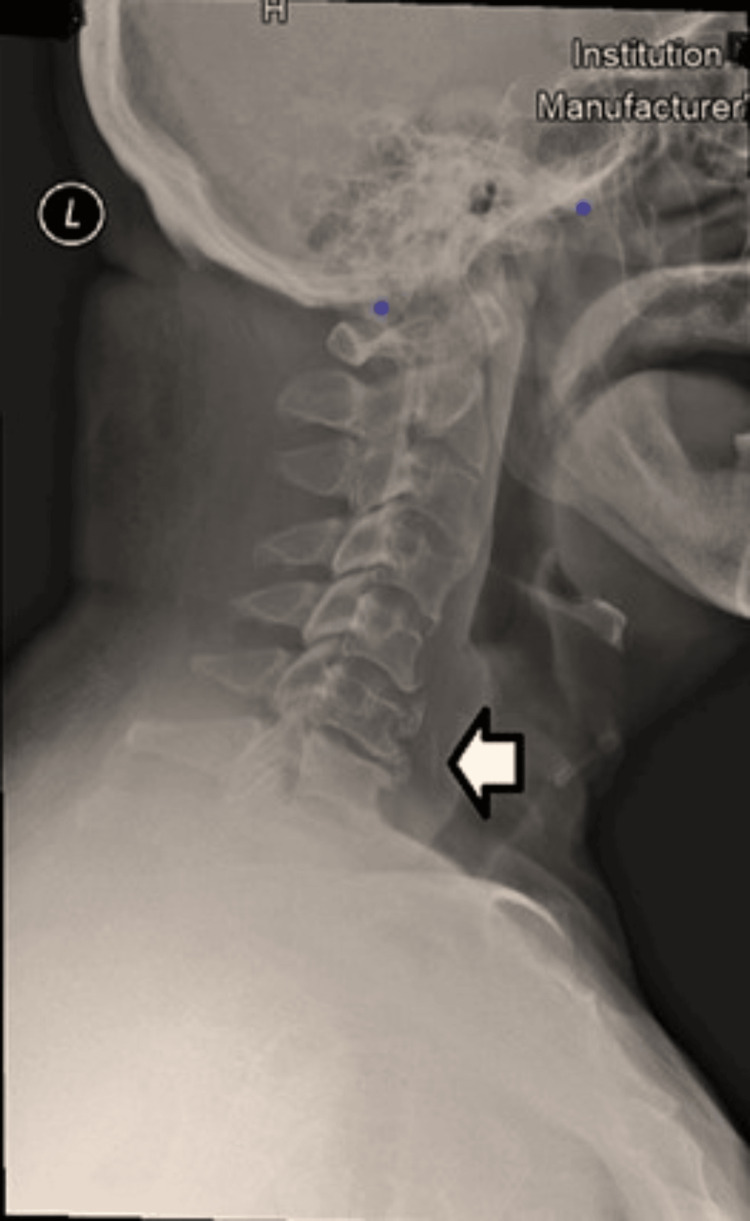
Plain lateral neck X-ray showing the fish bone at the level of the C6 vertebral body.
